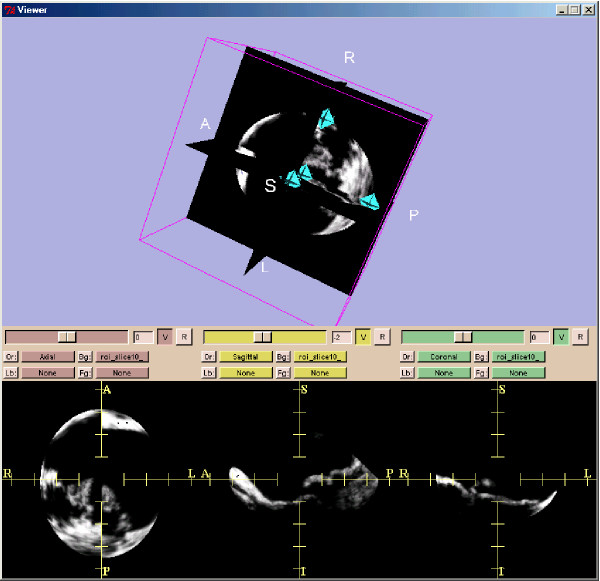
Ultrasound data after applying the elliptical region-of-interest (ROI). Ultrasound data of the same patient as in Figure 2 after applying the elliptical region-of-interest (ROI) defined by the four fiducials (above frame). The three planes for each spatial direction are shown in the lower three frames.
